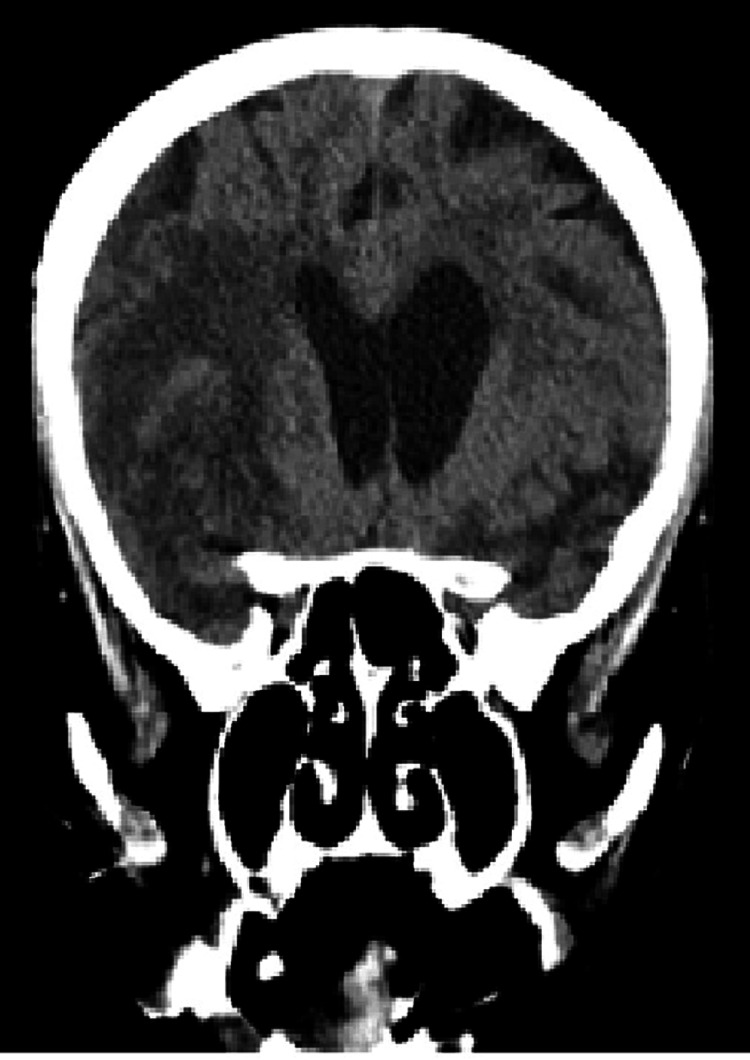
Coronal CT brain on day four of admission, demonstrating widespread vasogenic oedema in the right frontal, temporal, and parietal lobes. Moderate mass effect with effacement of the posterior right lateral ventricle with associated midline shift. Features of early hydrocephalus with distention of the left temporal horn are also demonstrated.
